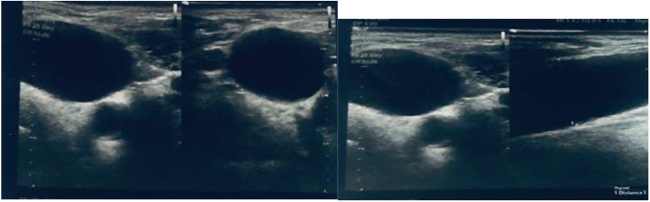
Ultrasound image of the mass showing fusiform dilatation of the right internal jugular vein, measuring 2.2 cm at its widest transverse diameter, which exceeds the normal range.
